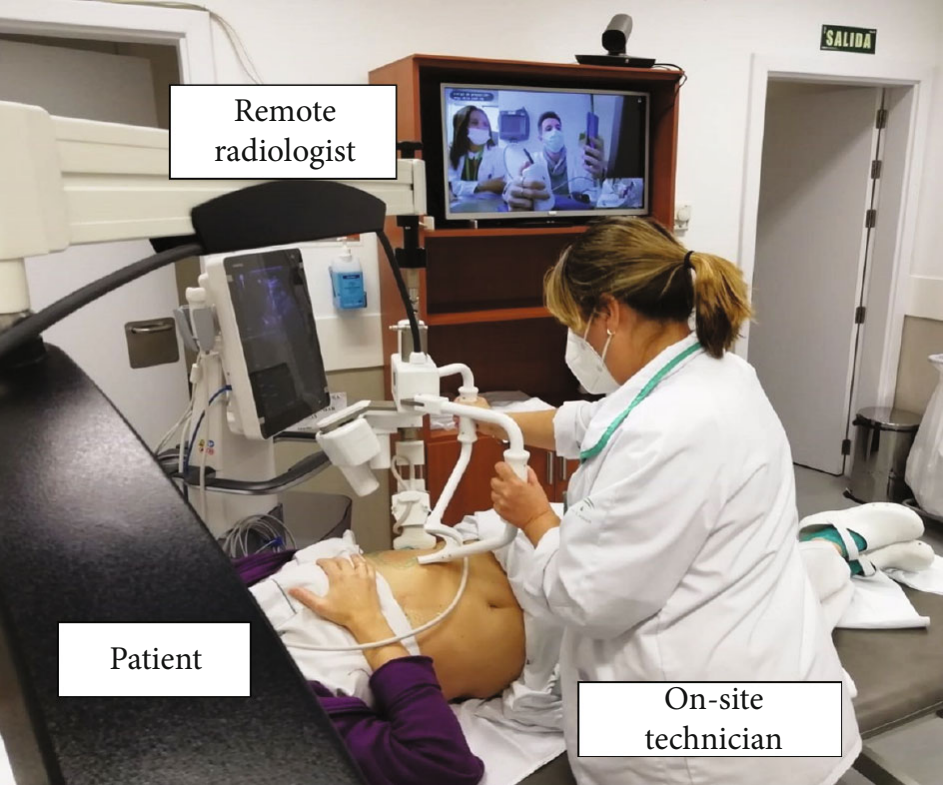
Telerobotic system used in the study (Melody, AdEchoTech). Technician placing the ultrasound probe with the patient in the supine position and on-screen remote radiologists performing telerobotic ultrasound.
